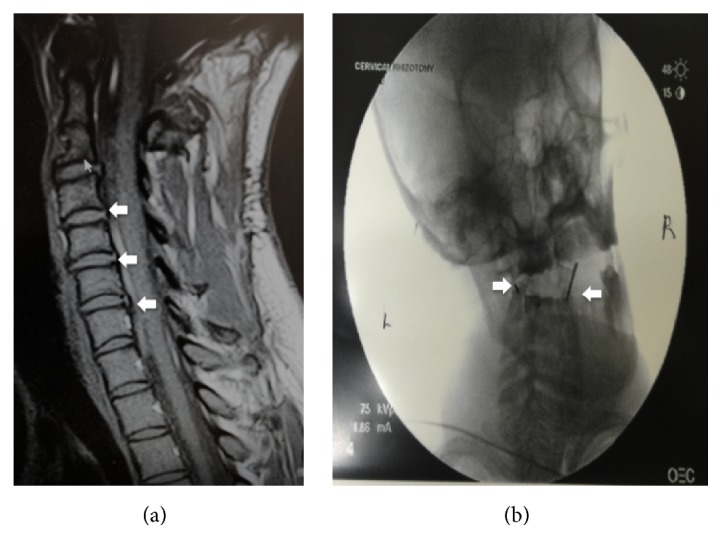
(a) MRI of the cervical spine showed degenerative changes observed at C2 (thin arrow). Multilevel disc desiccation at C2–C5 without disc height loss; C2-C3 and C3-C4 disc bulge with uncovertebral joint hypertrophy and spinal canal stenosis (fat arrows) were noted. (b) Open-mouth view of atlantoaxial joint confirmed bilateral intra-articular RFN needle placement (arrows) into the AA joint.
